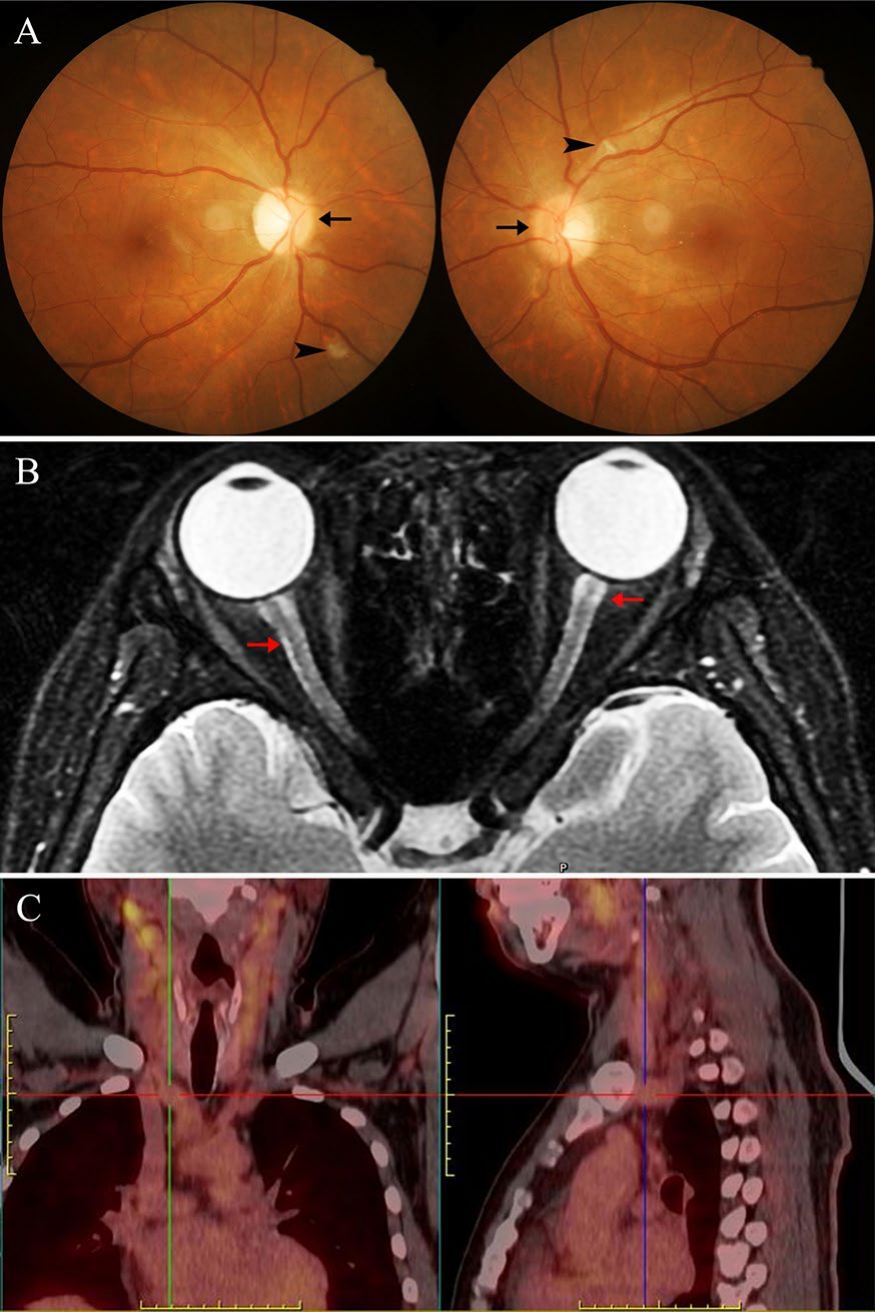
A 32-year-old man. A, Fundus photograph: bilateral disc edema (black arrow), retinal cotton wool spots (black arrowhead) presented on both eyes. B, Axial T2-weight MRI: a discrete T2-weighed hyperintense signal at both the optic nerve and sneath (red arrow). C, 18F-FDG-PET: multivessel disease through high accumulations of radioisotopes (SUVmax:3.7)
